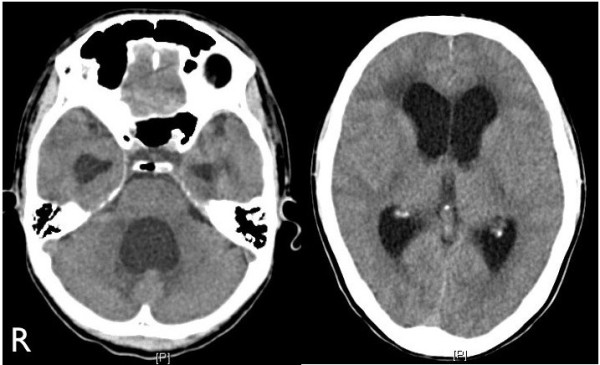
Computed tomography performed at admission showed remarkable hydrocephalus with dilatation of all ventricles, particularly the fourth ventricle.
